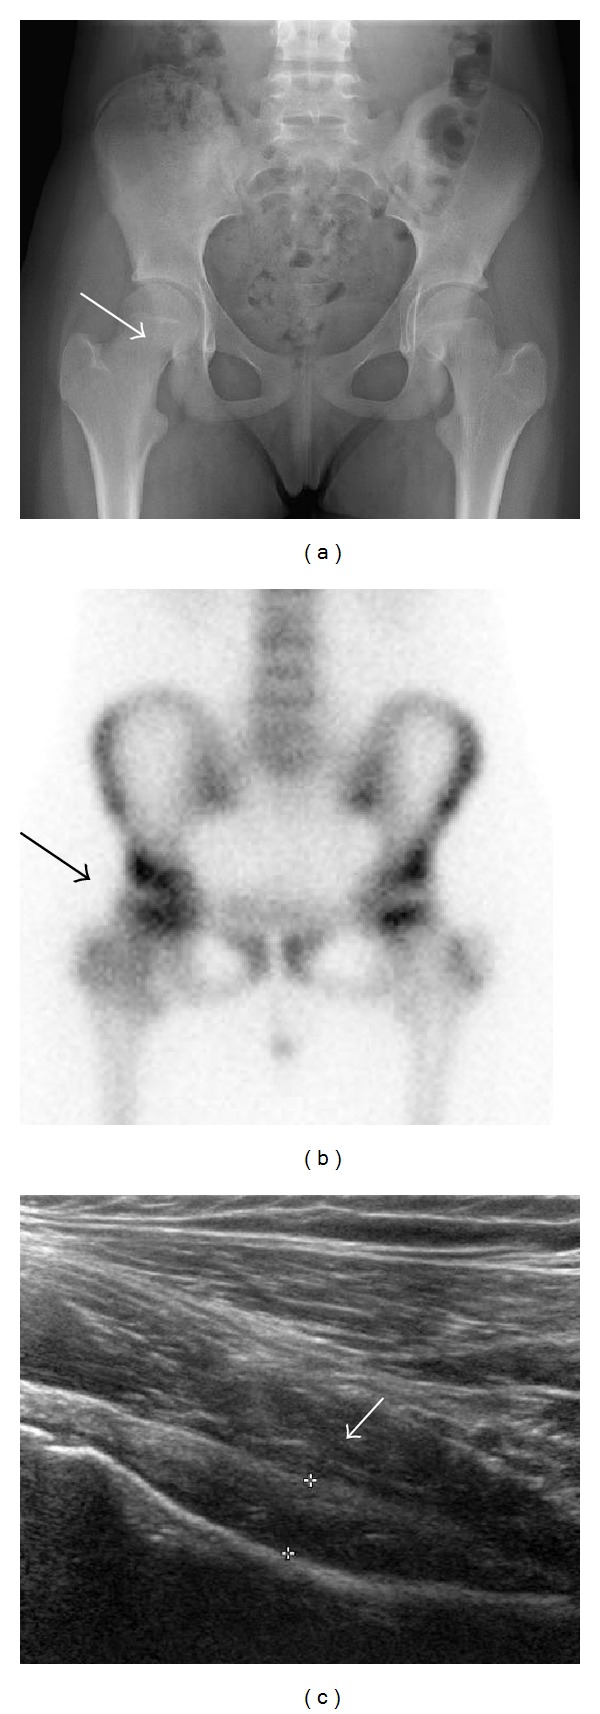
14-year-old girl with intra-articular osteoid osteoma of the right femoral neck. (a) Frontal X-ray of the pelvis, interpreted as normal. (b) Bone scan, demonstrating increased uptake of the right hip joint. (c) Hip ultrasound, longitudinal scan, showing synovial thickening of the anterior articular recess, right hip.
